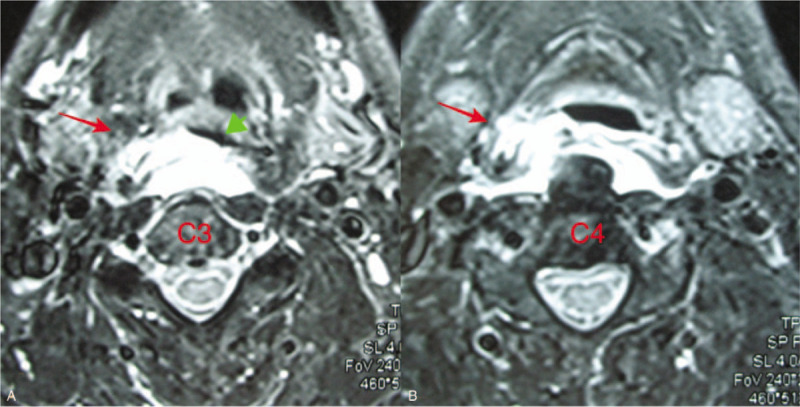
Axial MRI slices through C3 (A) and C4 (B) on T2-weighted images showed increased signal (arrow) between the posterior wall of the laryngopharynx and the cervical vertebral bodies. The pharyngeal cavity almost closed (arrowhead). MRI = magnetic resonance imaging.
